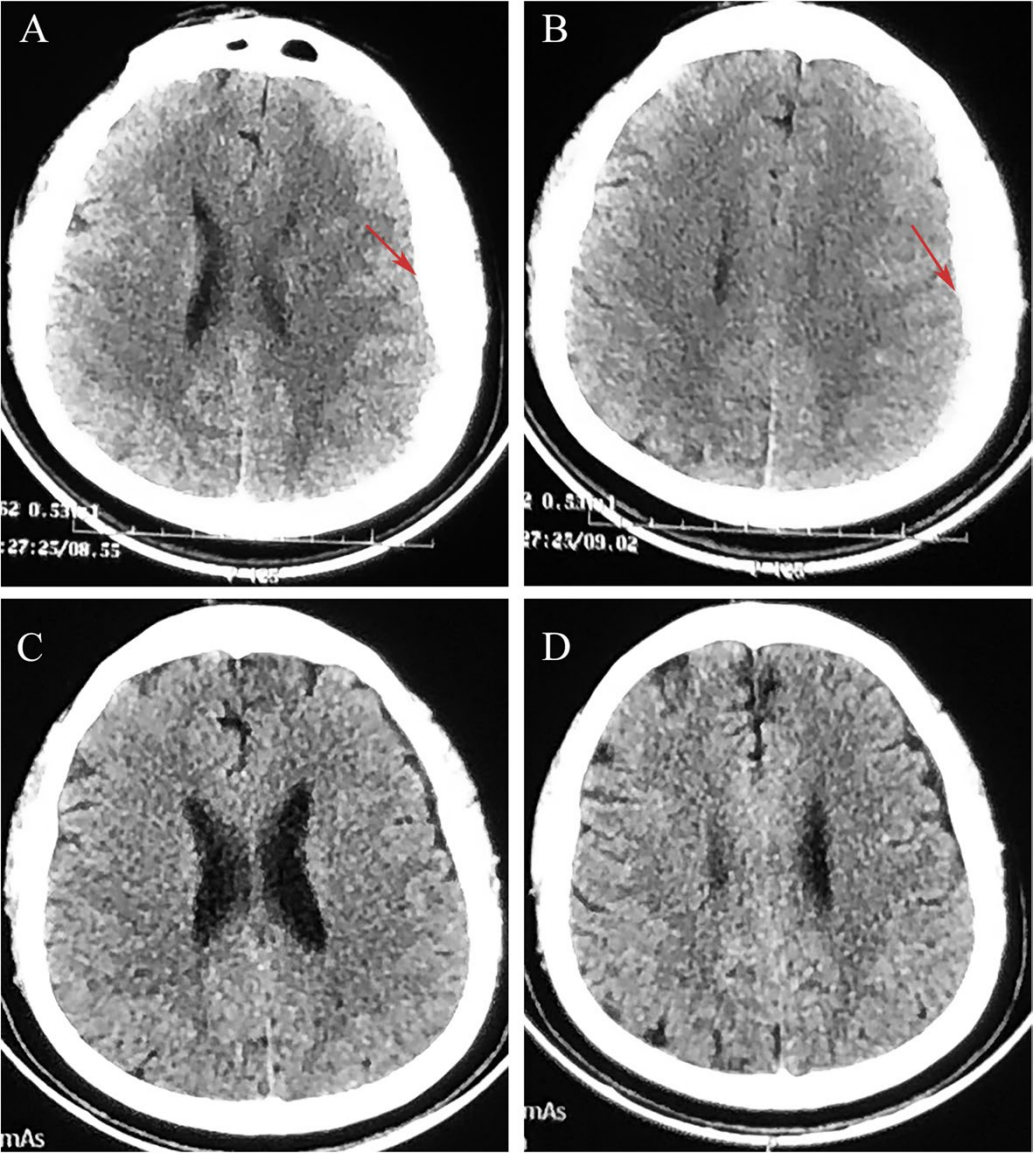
CT scan images for patient 2. Initial brain CT revealed ASDH (red arrows) in the left hemisphere mimicking skull thickening (A, B). CT scan revealed complete resolution of the hematoma after 2 months (C, D)
